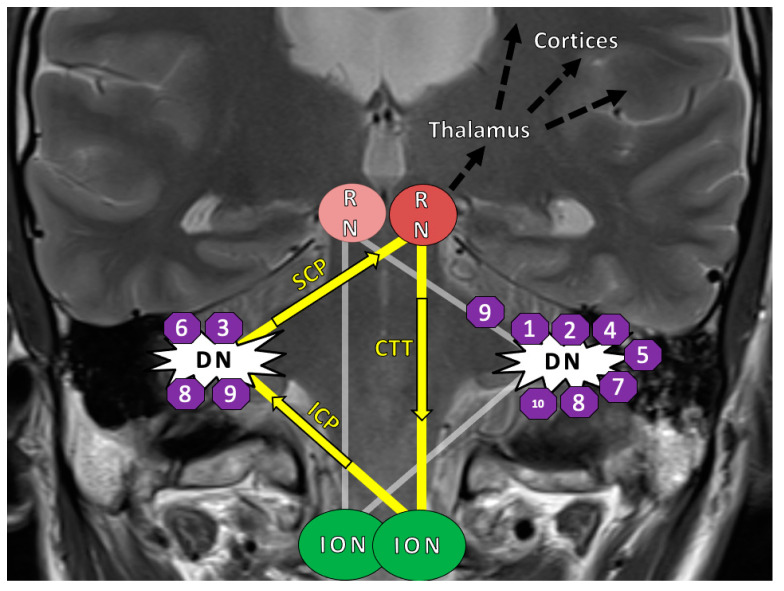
Disease model with lesion sites: The Guillain–Mollaret-triangle (yellow) and its unidirectional course of inhibitory fibers that ascent from the dentate nucleus (DN) to the contralateral red nucleus (RN) and then descend ipsilateral to the inferior olivary nucleus (ION). The bilateral overlapping Guillain–Mollaret triangles (yellow and grey) together form a “tilted star of David configuration” [5]. The numbers in octagons indicate the position of the operative lesions in Patients 1–10 of this study, who ultimately developed a HOD appearance on MRI. In bilateral lesions, octagons are shown on both sides. Fibers of the GMT, which is also called the dentato–rubro–olivary-pathway, overlap with dentato–thalamo–cortical pathways proceeding into both hemispheres (black arrows) [10]. Abbreviations: GMT = Guillain–Mollaret-triangle; HOD = hypertrophic olivary degeneration; SCP = superior cerebellar peduncle; ICP = inferior cerebellar peduncle; RN = red nucleus; CTT = central tegmental tract; DN = dentate nucleus; ION = inferior olivary nucleus.
